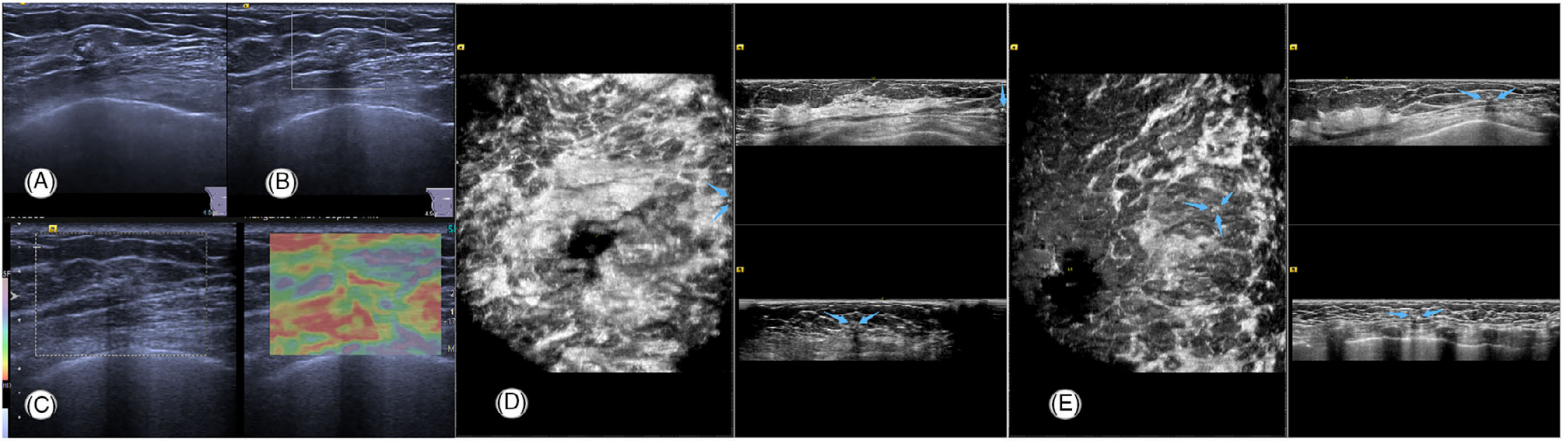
Ultrasonic finding of the right lesion by HHUS and automated breast US. (A) An irregular and indistinct hypoechoic nodule with calcifications detected in the 2‐o'clock position of the fat layer. (B) Color Doppler US reveals no internal color flow signals. (C) Elastography revealed a Tsukuba stiffness score of 2. (D, E) Anteroposterior (AP) view and medial (MED) view by AI‐breast US demonstrates a hypoechoic nodule 7 cm away from the nipple of the right breast (blue arrows), but only part of the lesion is shown on AP view
